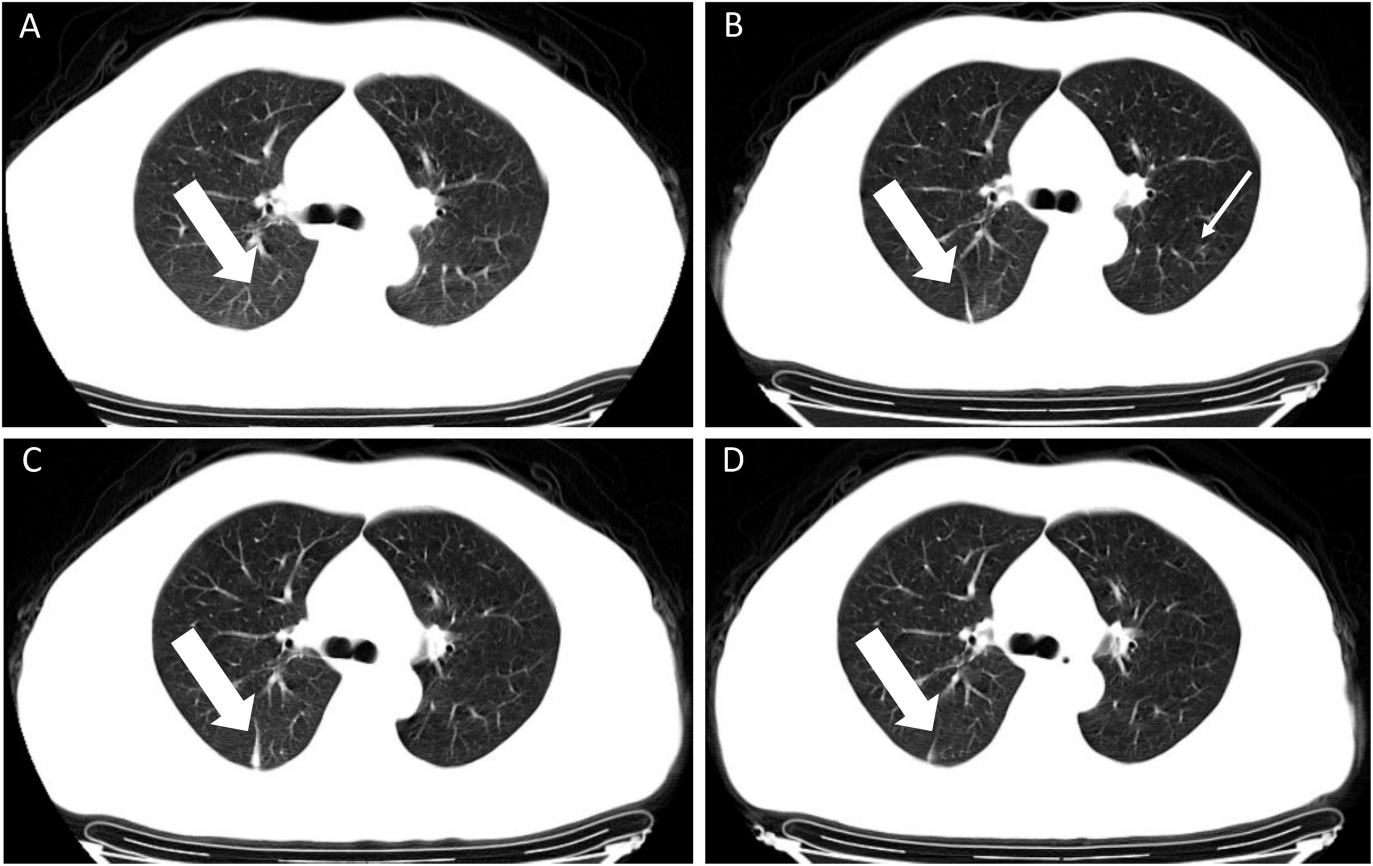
A 45-year old male with confirmed COVID-19 infection. Patient had a direct exposure history to Wuhan and the onset symptom of vomiting. (A–D) CT scan performed four times. Initial CT scan (performed on January 30 2020) showed no obvious abnormal CT findings (A). The next three times follow-up CT images showed a strip lesion in the right lower lobe (white thick arrow), first presented on second CT scan [performed on February 3 2020, (B)], enlarged on the third CT scan [performed on February 6 2020, (C)] and absorbed on the fourth CT scan [performed on February 9 2020, (D)]. An ambiguous lesion was shown in the upper left lobe [white fine arrow, (B)] and was absent in other images. The viral load (*Ct* value) was 22.53.
